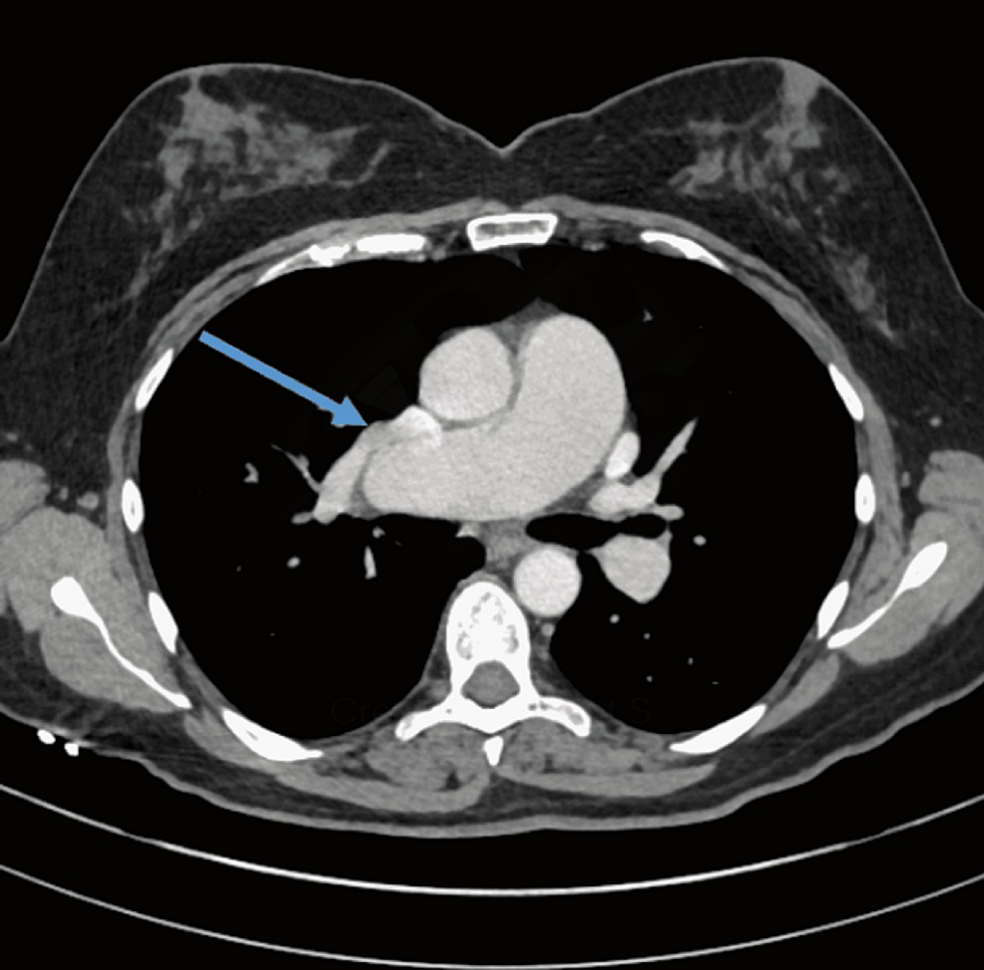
Chest CT scan with contrast showing PAPVR with the right upper and middle lobes draining into the right-sided SVC Arrow: right upper lobe vein draining in SVC PAPVR - partial anomalous pulmonary venous return; SVC - superior vena cava
